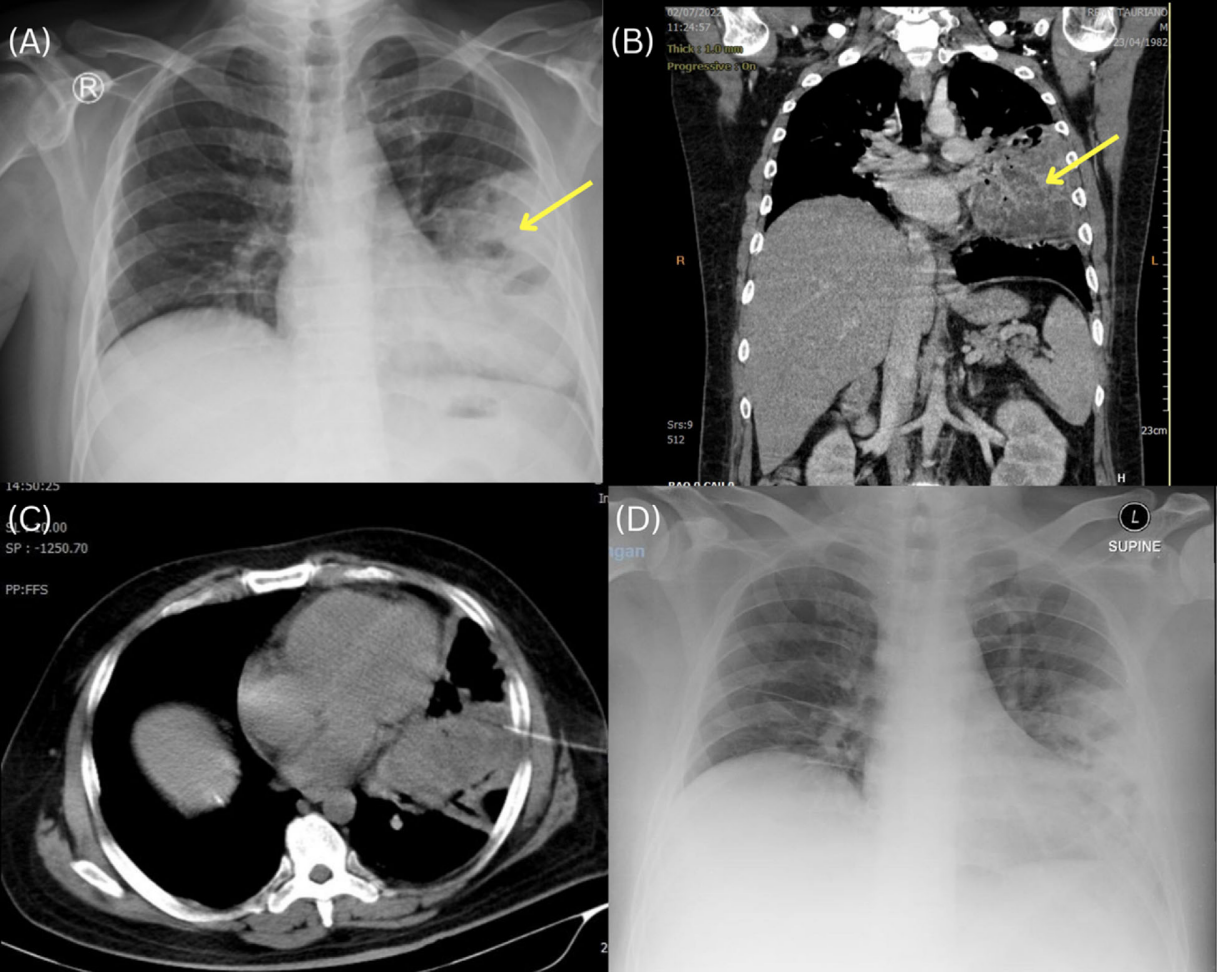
(A) The initial chest x‐ray demonstrates left paracardial consolidation possible pneumonia or mass (arrow). (B) Chest CT scans with contrast, coronal view shows extensive consolidation in the left paracardial with air‐filled areas indicating lung abscess, and suspicious neoplasm (arrow). (C) CT‐guided transthoracic biopsy. (D) Chest x‐ray 1 month after evaluation shows consolidation reduced, relatively thinner.
